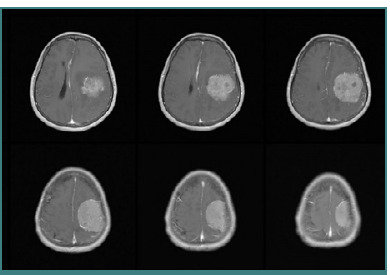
CT scan showed compressed sulcus and gyrus, peritumoral edema, midline shift
